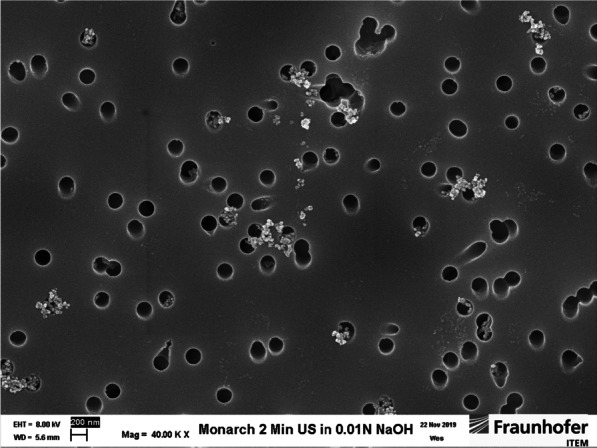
Preparation of Monarch® 1000 suspension in 0.01 NaOH–2 min ultrasonic treatment
